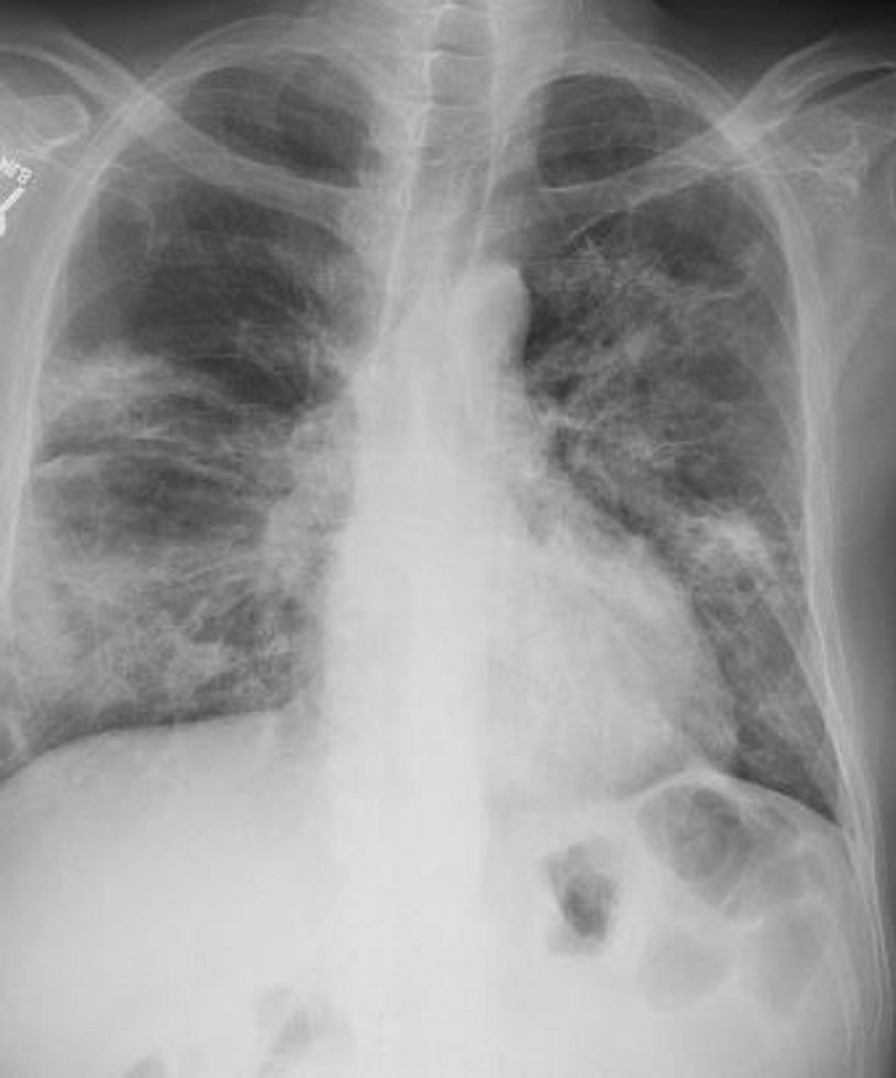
Case 8. Figure 2. Chest X-ray. Multifocal interstitial and patchy alveolar airspace opacities noted throughout the mid right lower lung and mid left lung
